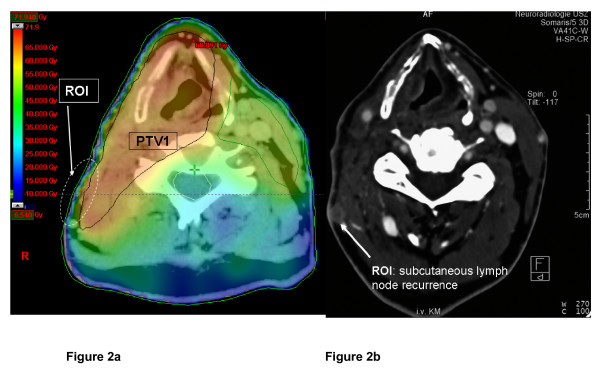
Superficial nodal recurrence (ipsilateral dorsolateral node) in a patient with a pT2 pN2b supraglottic larynx carcinoma. Preoperative diagnostic images did not show any suspicious superficial nodes, nor were any enlarged nodes visible in the postoperative planning computed tomography a: IMRT treatment plan, PTV1 (black line): 'build up' effect in the skin/subcutaneous region of interest (ROI, white dotted line) which was not intended to be included into the PTV1. b: posttreatment follow up computed tomography scan revealed a superficial lymph node metastasis (ROI), located in the former 'build up' area
